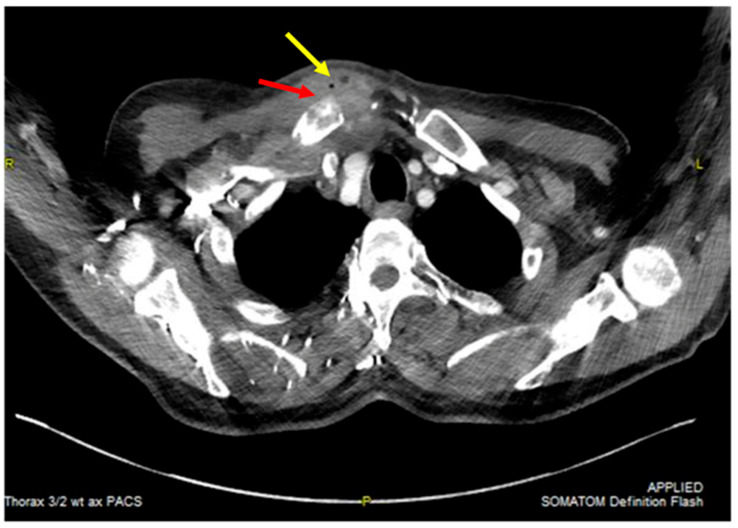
Axial CT scan of the chest showing an abscess in the right sternoclavicular joint region with expansion into adjacent soft tissues. Air inclusions (indicated by the yellow arrow) are visible, along with signs of osteomyelitis in the surrounding bone structures (indicated by the red arrow), indicating an advanced stage of infection.
